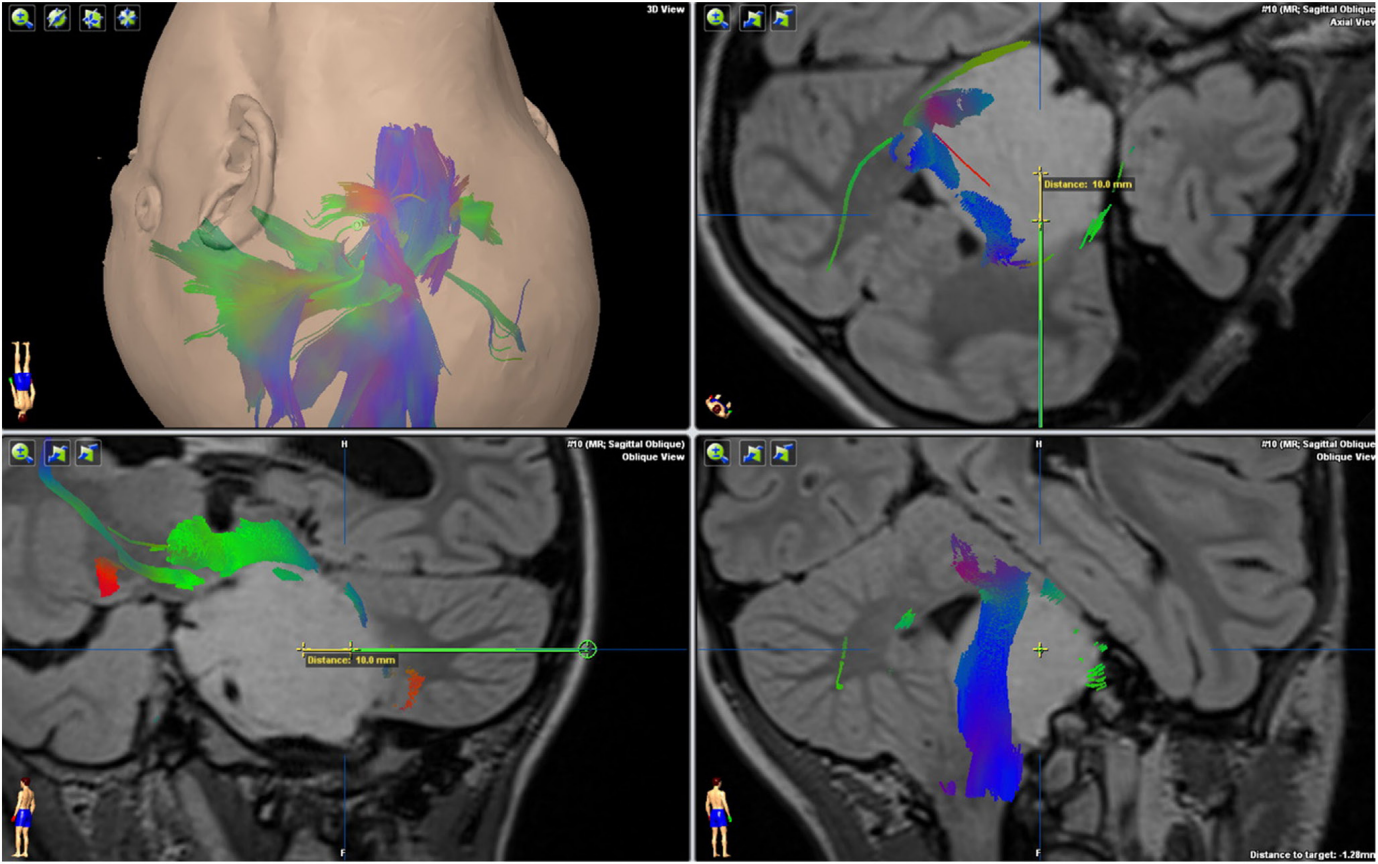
Magnetic resonance imaging T2 FLAIR 3D sequence, with DTI tractography fusion showing the relationship between the tumor and major brain tracts. The green trajectory of the biopsy avoids the corticospinal tract ending in the biopsy target. The yellow segment notes the extra depth (10 mm) of the infusion cannula tip.
